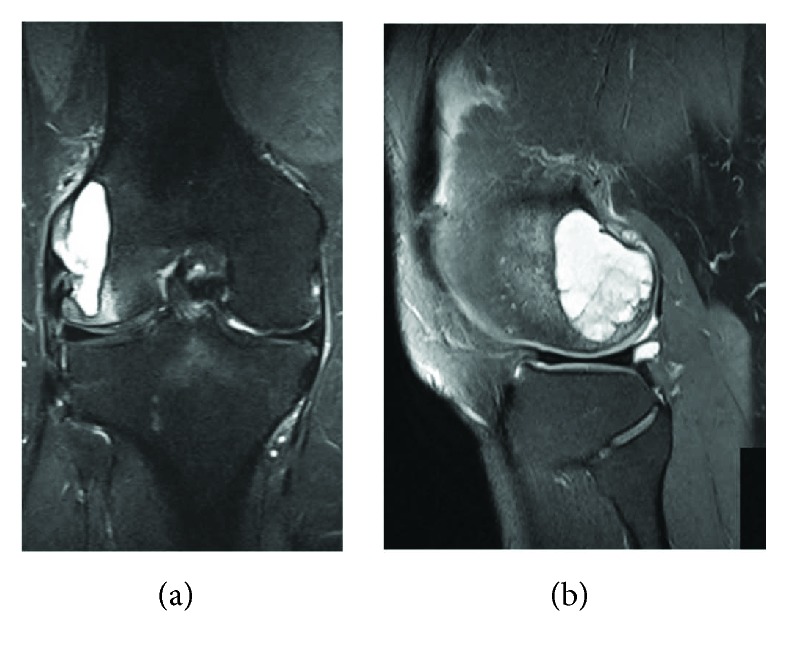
T2-weighted coronar (a) and sagittal (b) MR images of the right knee showing a giant cystic lesion of the lateral femoral condyle.
